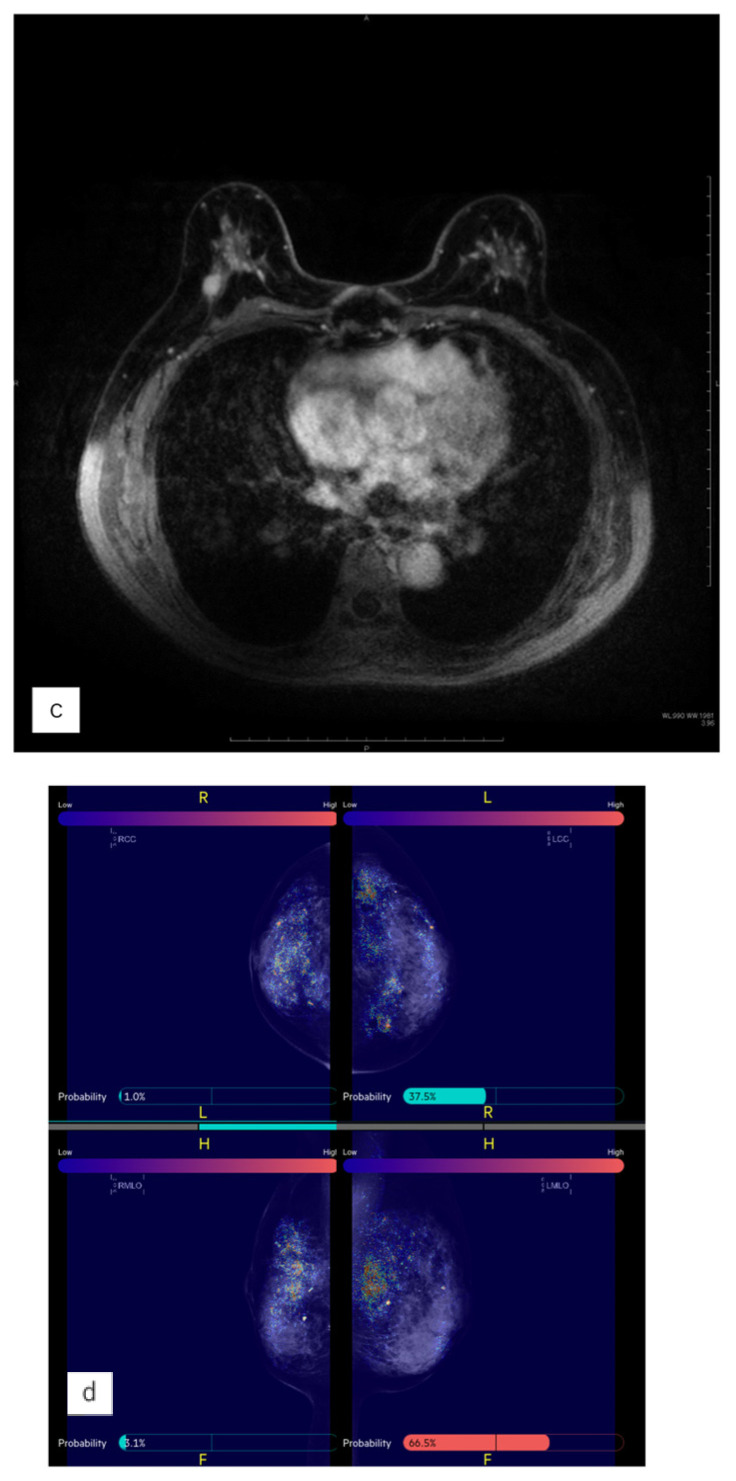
Representative case (case 7). (a) MG. (b) US. (c) MRI. (d) AI diagnosis. A 63-year-old woman had left-sided breast cancer. Two years later, she was diagnosed with right breast cancer. It was T1c, triple-negative breast cancer. (a) A mass was found in the right upper quadrant via mammography (MG) and diagnosed as a breast imaging reporting and data system (BI-RADS) Category 4. (b) Ultrasonography revealed a hypoechoic mass in the right upper outer quadrant. (c) Magnetic Resonance Imaging revealed a contrast-enhanced mass in the left upper outer quadrant. (d) The artificial intelligence system detected no malignancy. The mass visible on MG was not seen on MG a year earlier. Although the mass was of the same density as the background mammary gland, the radiologists found it to be possibly malignant upon comparison and reading. The malignancy percentage is shown on the left and right sides, respectively (CC: right 1.0%, left 37.5%, MLO: right 3.1%, left 66.5%).
